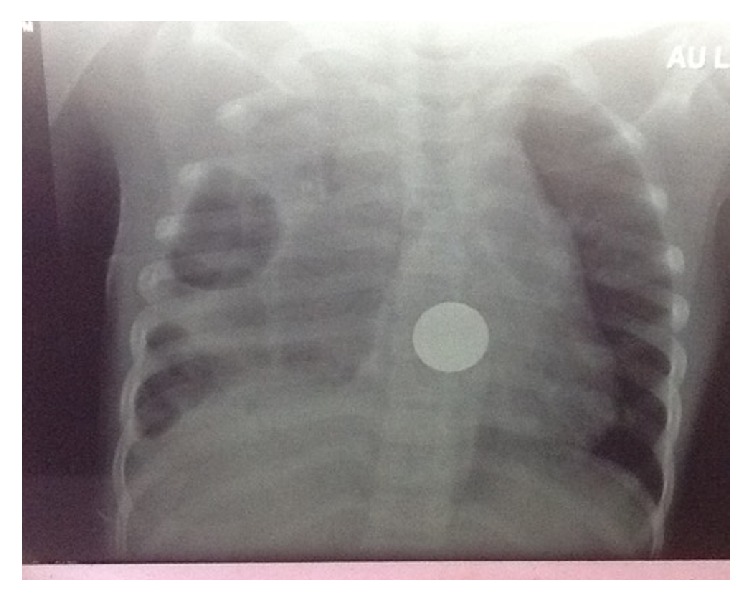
Chest X-ray of the same girl 12 days after admission, loculated empyema, and foreign body at almost the same position.
